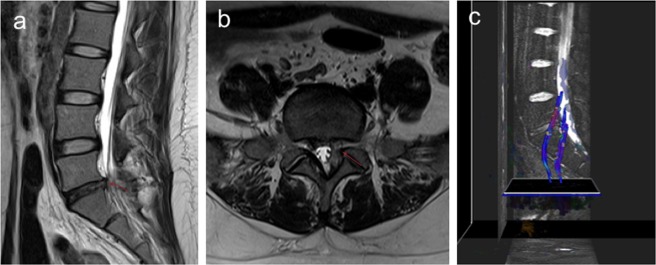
Lumbar disc MRI and lumbosacral nerve root DTI scan at 90 days post-surgery. (a) is the patient’s MRI sagittal position 90 days post-surgery, the posterior longitudinal ligament is reattached, and the “grape skin” phenomenon has basically disappeared. (b) is the patient’s MRI axial position 90 days post-surgery, showing the spinal canal is well dilated. (c) is the nerve root reconstructed by DTI 90 days post-surgery, and the compressed nerve root microstructure is fully restored, comparable to the uninjured side.
